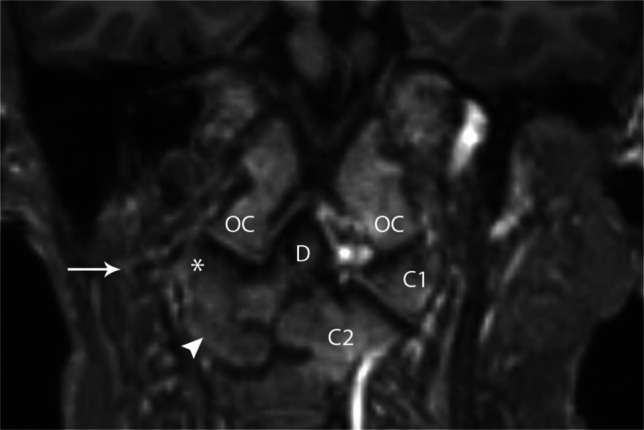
A Sagittal T2 MRI and (B) sagittal CT in a 13-year-old male demonstrate hypertrophied os odontoideum (*asterisk*) separated from the remainder of C2 by a cleavage plane (*arrowhead*). B Subsequent CT demonstrates posterior translation of the os odontoideum (*asterisk*) compatible with instability. Note spinal canal stenosis with cord signal abnormality (*double arrow*)
